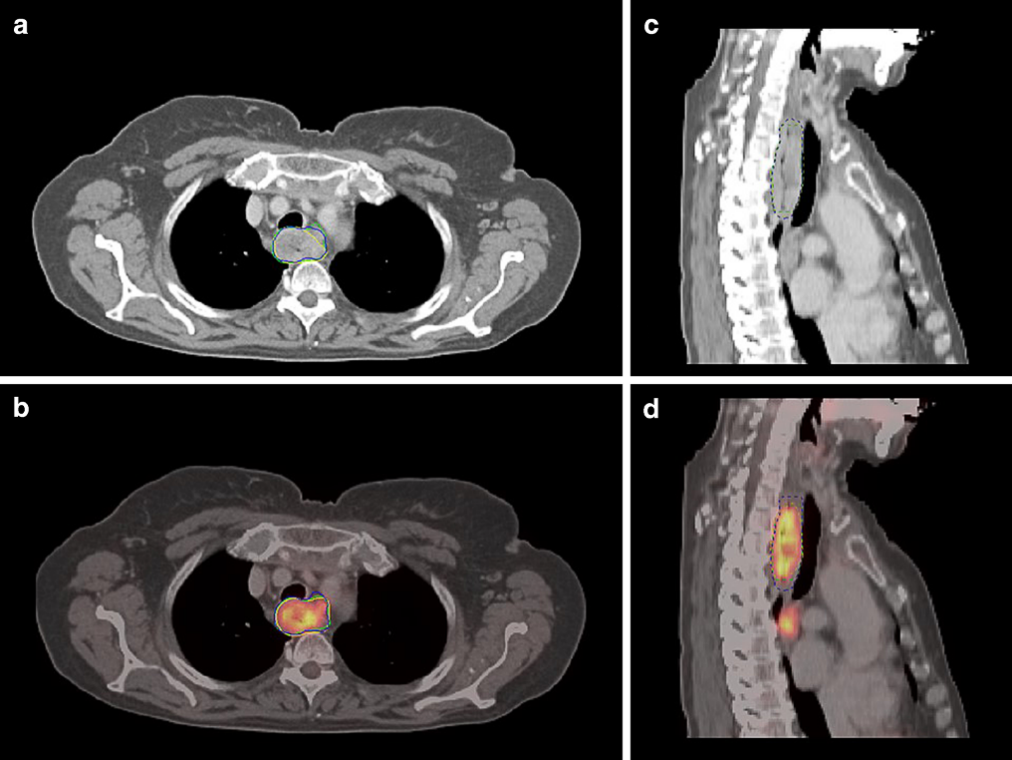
Manual gross tumor volume (GTV) delineation by three observers (*yellow*, *blue*, *green*) a on CT alone axial and b sagittal; c GTV delineation on fused PET/CT axial and d sagittal
